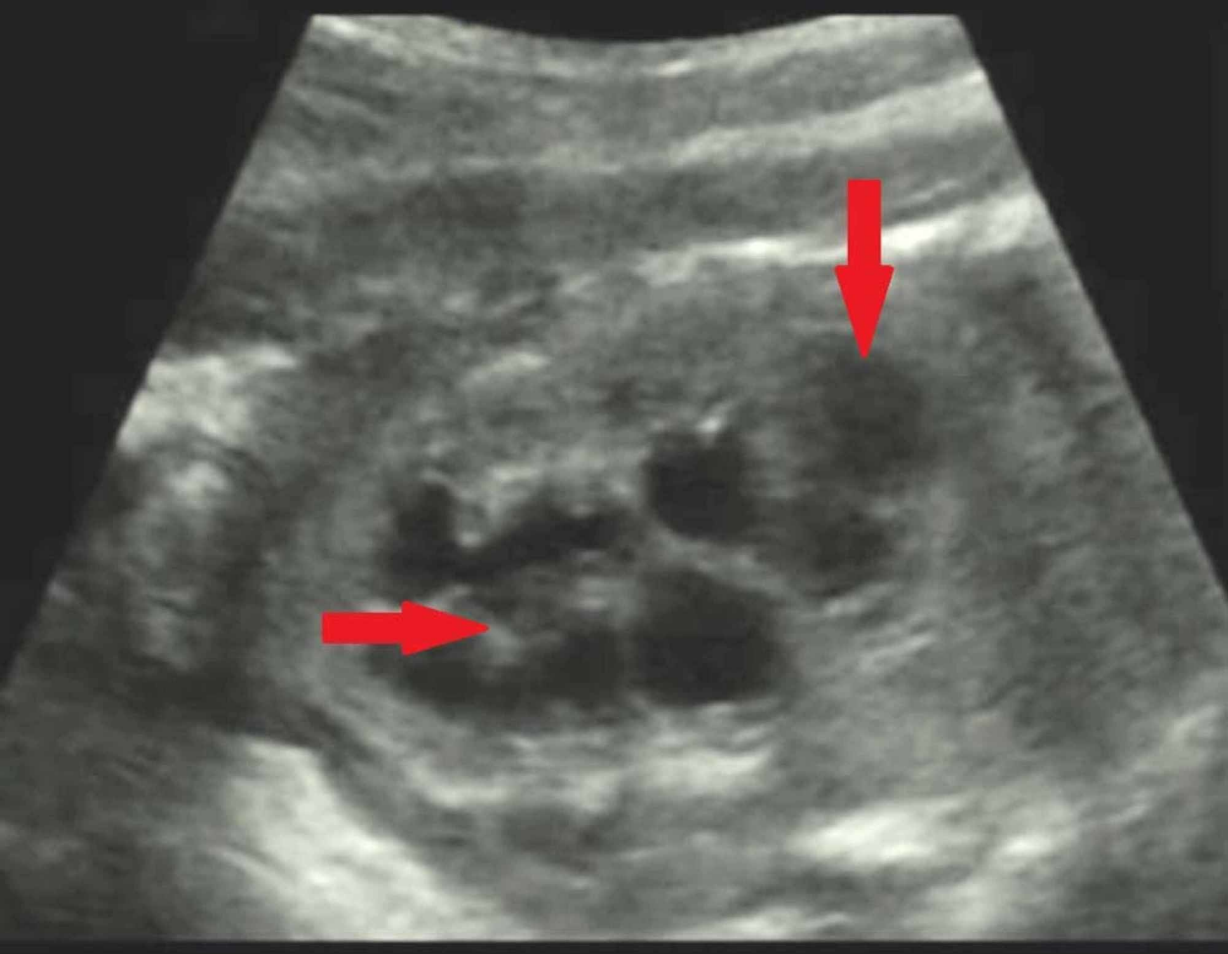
Ultrasound showing left dysplastic kidney showing multiple cysts
